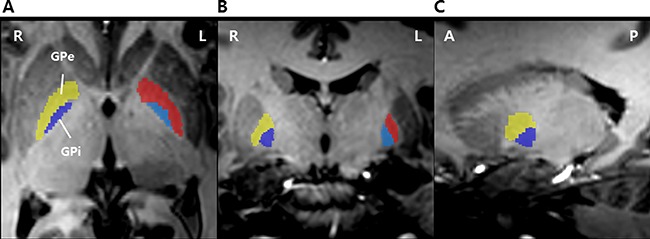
Volume segmentation of the GPi and GPe in the 7-Tesla MRI image. Volume segmentation of the GPi and GPe on the transaxial (*A*), coronal (*B*), and sagittal planes (C). Yellow overlay: manual labeling of the right GPe; red overlay: manual labeling of the left GPe; navy overlay: manual labeling of the right GPi; and sky-blue overlay: manual labeling of the left GPi. A: anterior; GPe: external globus pallidus; GPi: internal globus pallidus; L: left; P: posterior; R: right.
